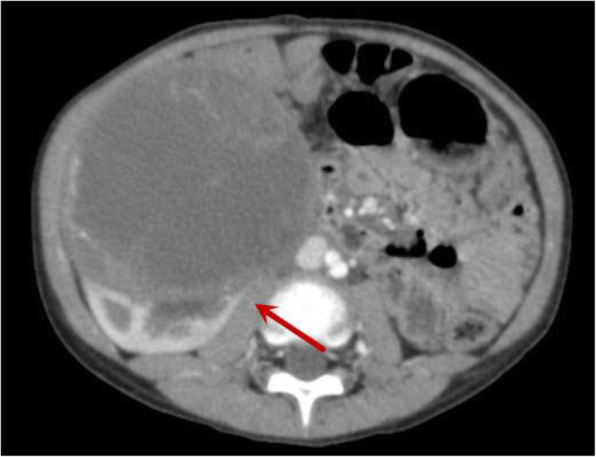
CT abdomen showing right Wilms’ tumor with claw sign (red arrow)
